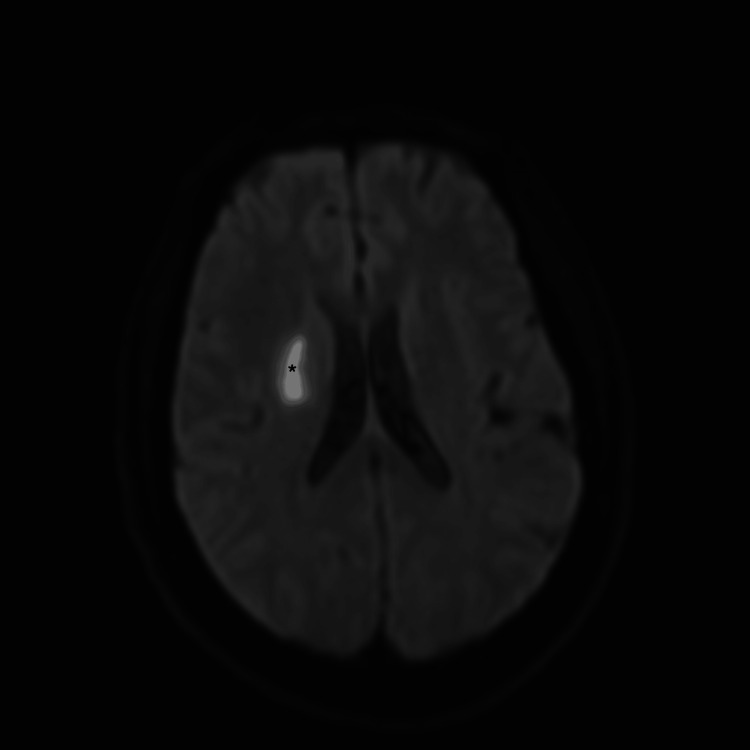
DWI MRI (B1000) revealing a deep MCA ischemic stroke. *Deep right ischemic stroke MCA, middle cerebral artery
